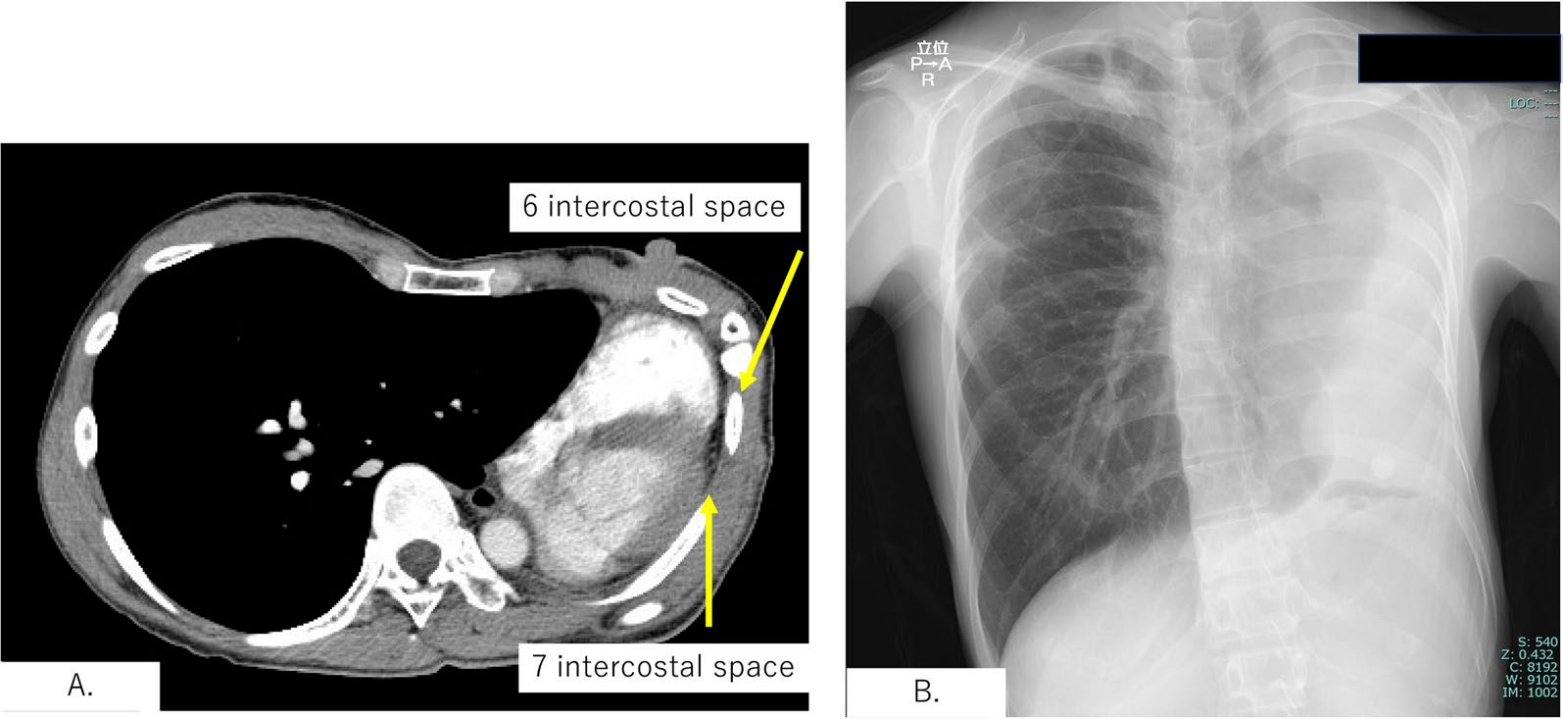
A Left thoracic cavity, previously vacant, no longer existed as the pericardial surface had attached to the thoracic pleura. Since the 5th intercostal space was accessed during the left pneumonectomy, we chose the sixth intercostal space for the thoracotomy. B X-ray also revealed that the left thoracic cavity had nearly disappeared due to mediastinal shift, with only a small gap between the chest wall and the pericardium anticipated during thoracotomy
